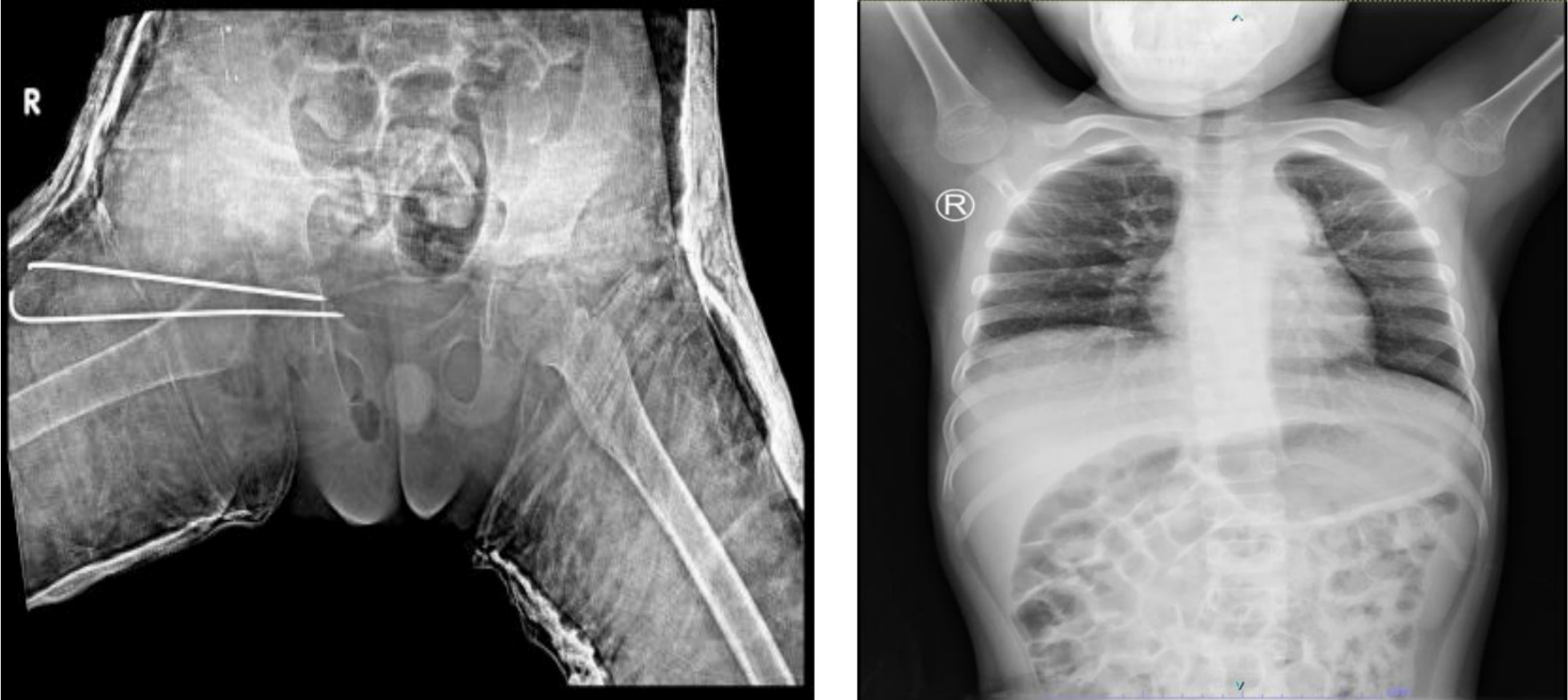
Postoperative pelvic x‐ray showing internal fixation using two smooth pins extending from the proximal right femur to the superior pubic ramus. The fixation appears stable with no evidence of loosening or malalignment (Left). Follow‐up chest radiograph obtained 2 months after initiation of anti‐tuberculosis therapy demonstrates marked resolution of bilateral patchy infiltrates, indicating early pulmonary improvement and good systemic response to treatment (Right).
